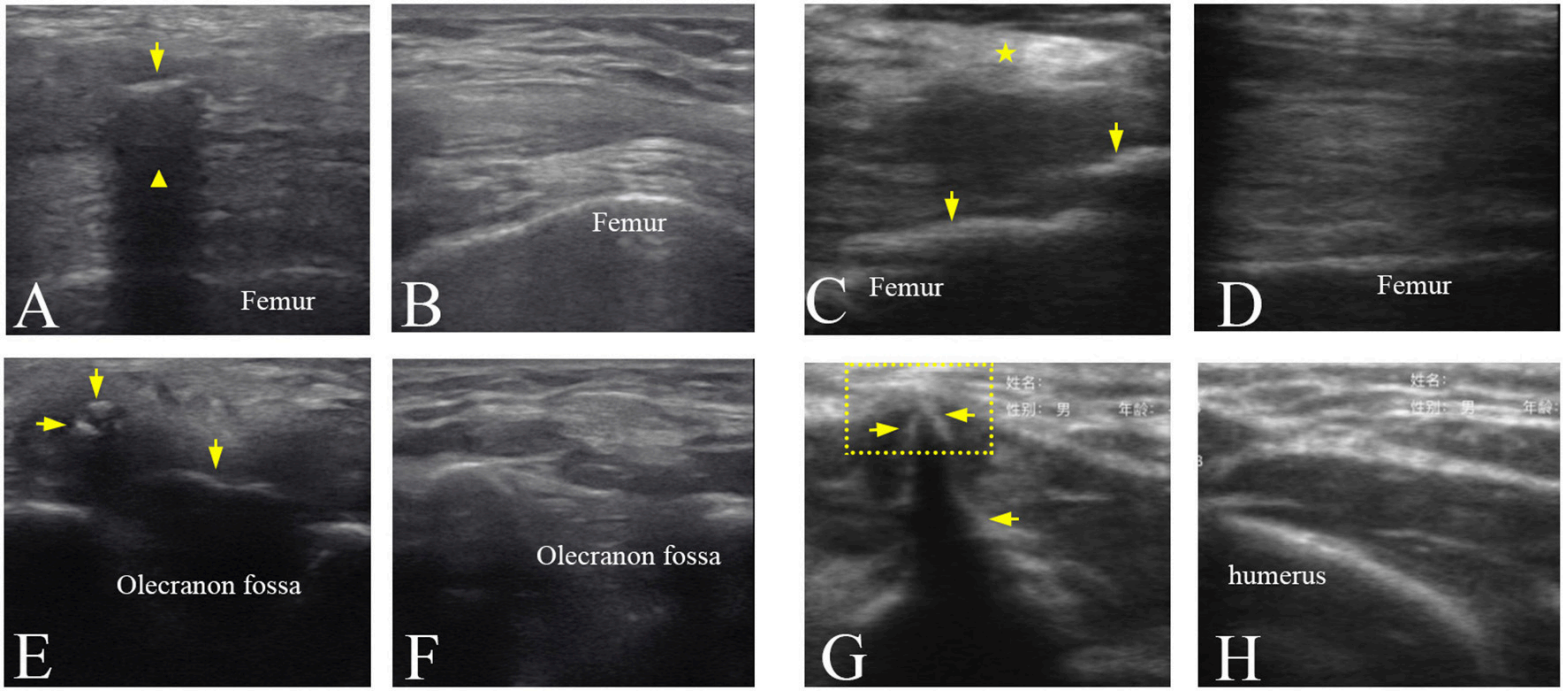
Ultrasound images of the mature HO in the different joints. (A) Ultrasound image of the left knee joint of participant No. 3 showing that HO (arrow) with an acoustic shadowing (
